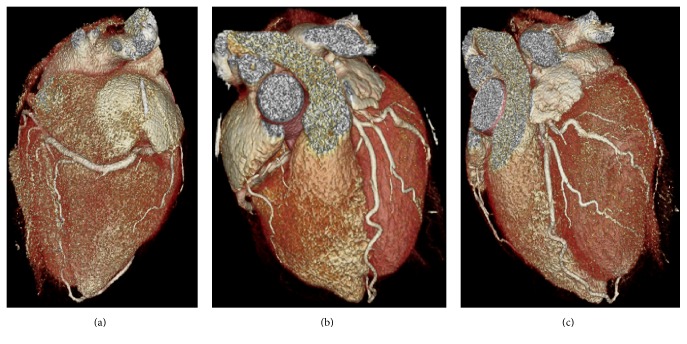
Coronary CT views: (a) left ventricle being supplied by branches of the RCA, (b) LAD in the interventricular groove, and (c) absent left circumflex artery.
